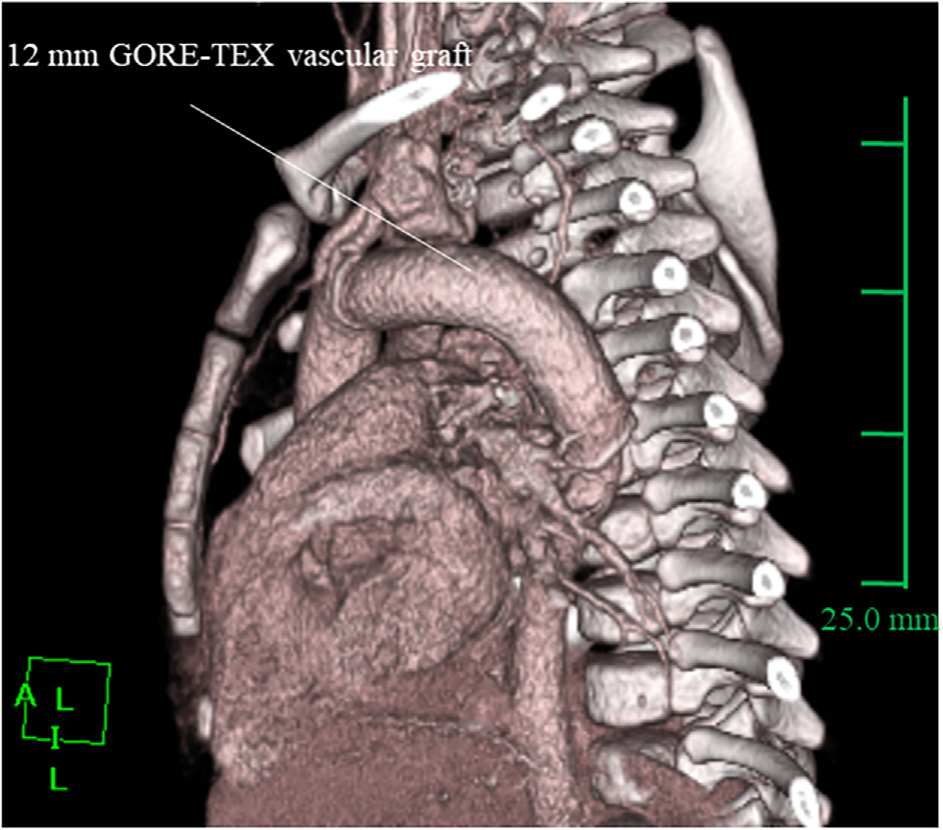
Postoperative 3-dimensional computed tomography image showing smooth interposition graft.
